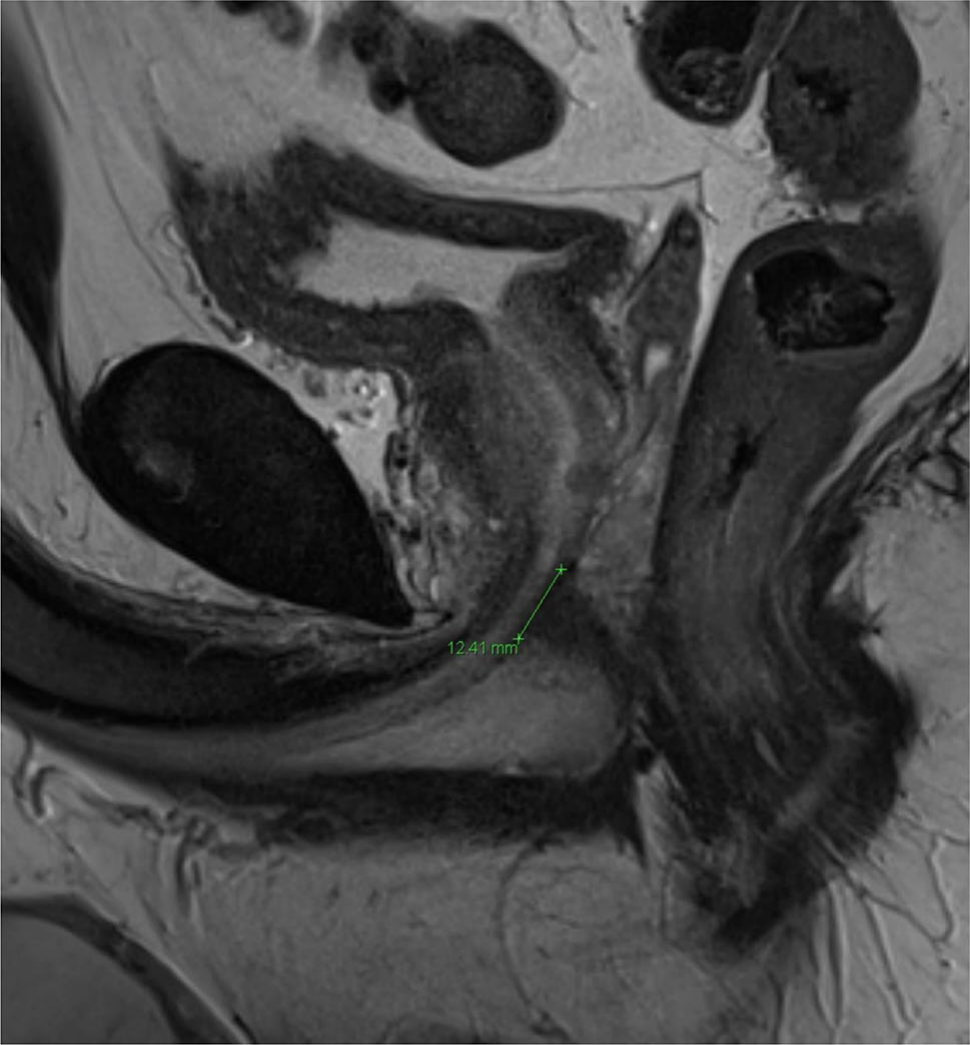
Urethral sphincter measurement in sagittal dimension in a multiparametric MRI prior to radical prostatectomy
